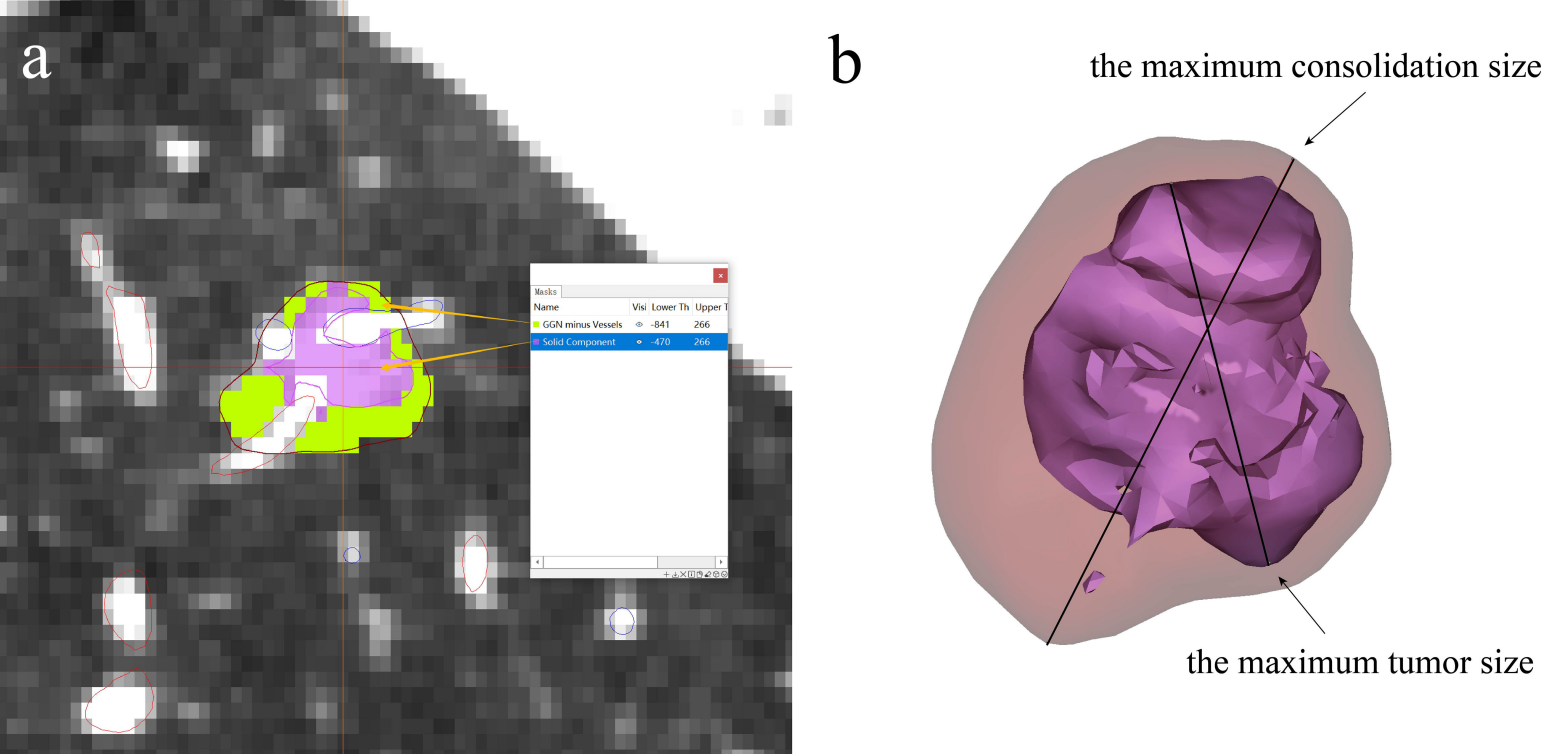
3D technology in lung nodule imaging diagnosis. (A) Nodule is divided into solid and ground glass component according to CT value, (B) CTR value can be obtained by calculating the maximum consolidation size to the maximum tumor size.
